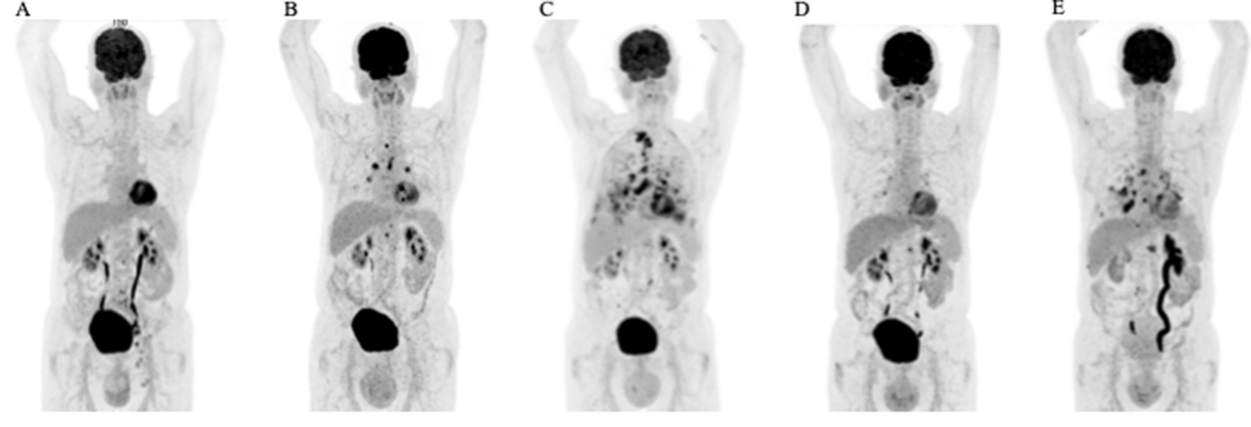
Therapeutic efficacy assessed by FDG-PET/CT scans. Coronal maximum intensity projection images (A) at the initial presentation, (B) following recurrent disease after radiation therapy (C) after lapatinib treatment (D) best response during trastuzumab and carboplatin treatment, and (E) disease progression after 10 cycles of trastuzumab and carboplatin.
